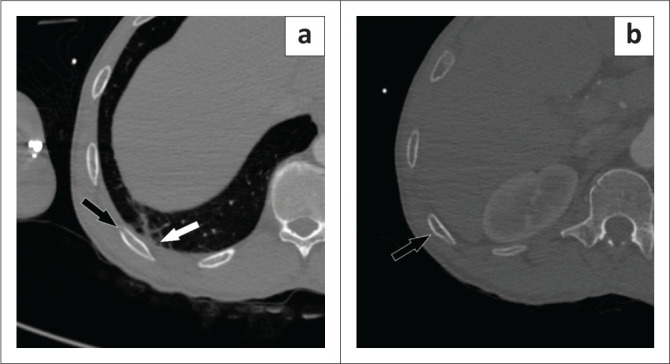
Axial bone reconstruction of a CT (a and b) of the chest demonstrating subtle buckle-type fractures of the 10th and 11th ribs (black arrows) associated with a small lung contusion (white arrow).
